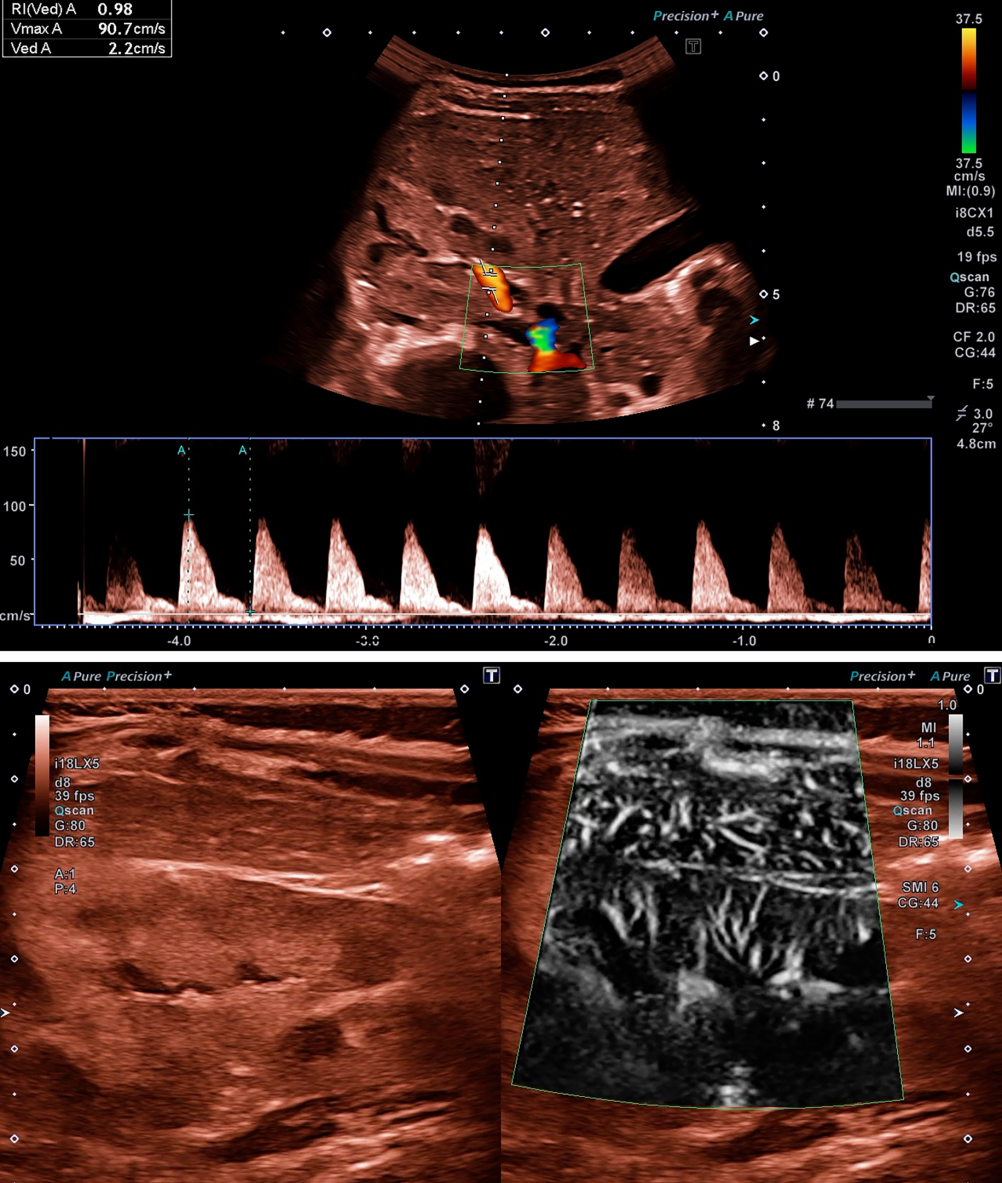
(A) Ultrasound examination of renal perfusion at disease onset with evidence of a significantly increased value for RI of 0.98 in a 10-month-old girl with STEC-HUS. (B) Reduced renal tissue perfusion in SMI mode, which is atypically even lower than the overlying hepatic tissue perfusion, in the same patient as in (A). Video presentation in the Supplementary Material Video S1.
